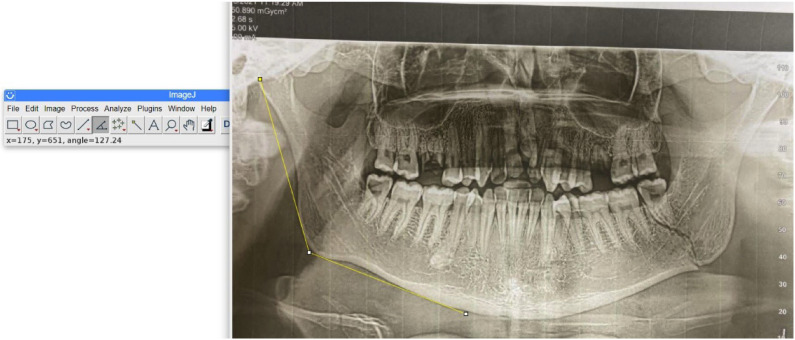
Measuring the gonial angle on digital panoramic radiograph using Image J program.
